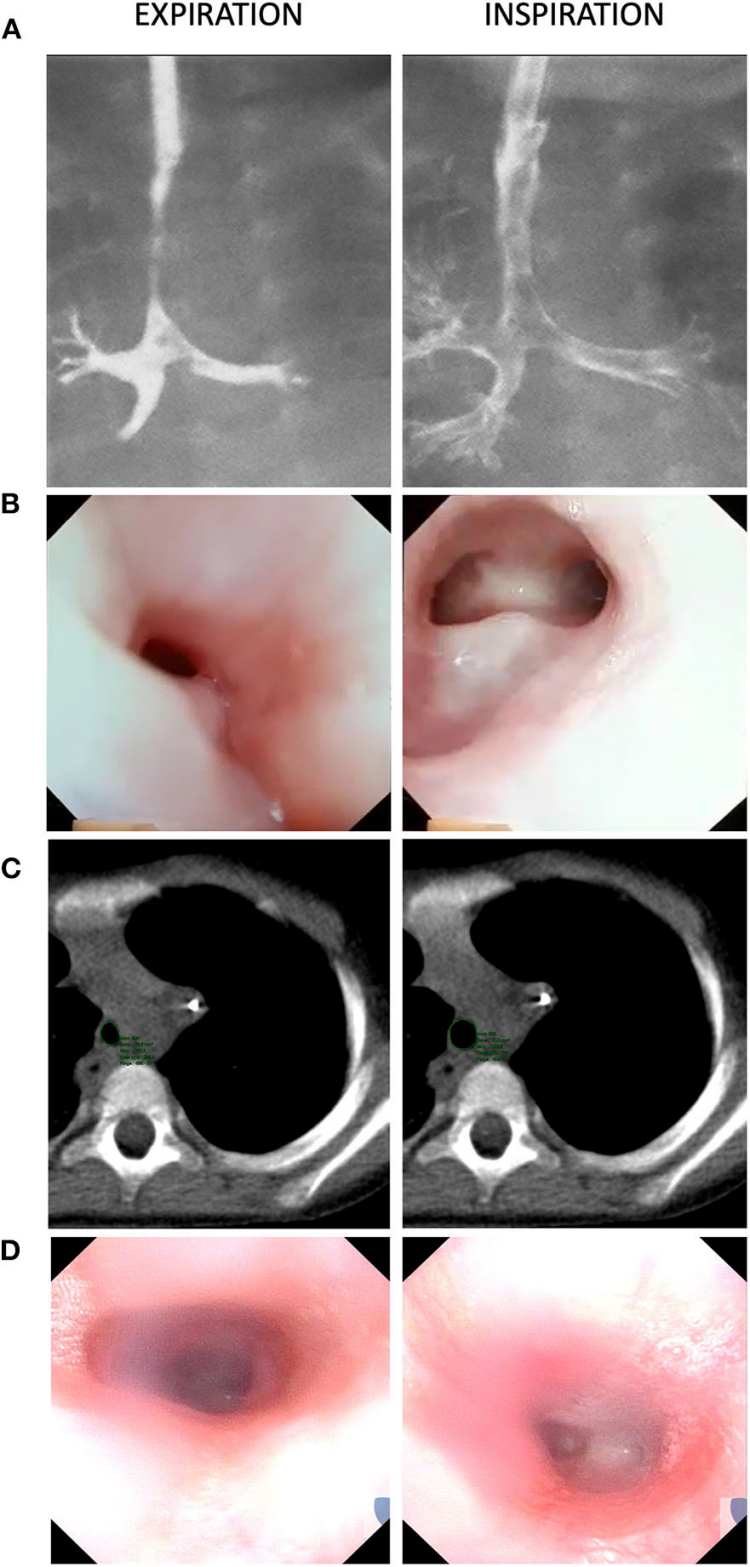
Expiratory airway collapse of the neo-tracheal segment, as observed via: (A) Tracheobronchography (Day 40). (B) Tracheoscopy (Day 865). (C) Dynamic computed tomography (CT) scan (Day 870). (D) Tracheoscopy (Day 1,798). Both tracheobronchography and tracheoscopy (images A,B), comparing expiration and inspiration frames, allow to appreciate esophageal-like appearance of the unsupported segment (due to the absence of cartilaginous rings), as also confirmed at CT scan (image C). At tracheobrochography (image A—inspiration frame), it is possible to appreciate a sort of diverticulum, just above the unsupported segment, which represents the site of separation of the proximal esophageal portion from the common segment. Similarly, in tracheoscopy views (images B,D—inspiration frames), a fovea on the posterior neo-tracheal wall, just above the carina, results from the separation of the distal esophageal portion. The most recent tracheoscopy (image D) confirms the absence of tracheal collapse 2 years after external stenting surgery.
